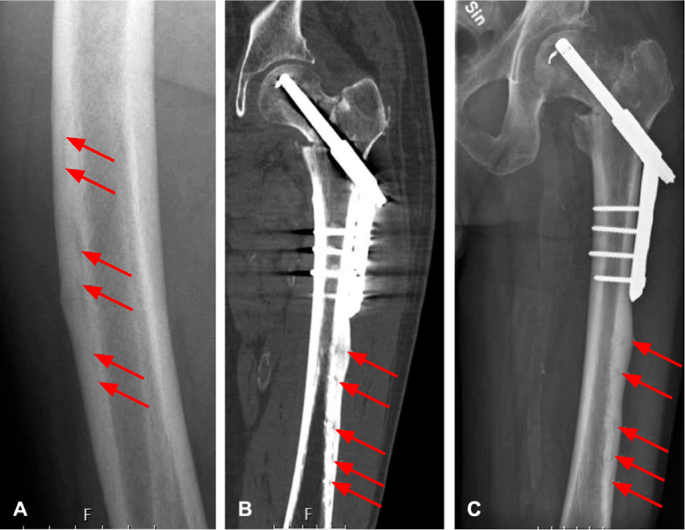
A. Cortical irregularities in proximity to mid-shaft incomplete atypical femur fracture; in Case 1. B and C. Case 3 was treated with a dynamic hip screw plate for an intertrochanteric femur fracture. On the postoperative radiograph, multiple cortical lesions are evident.
